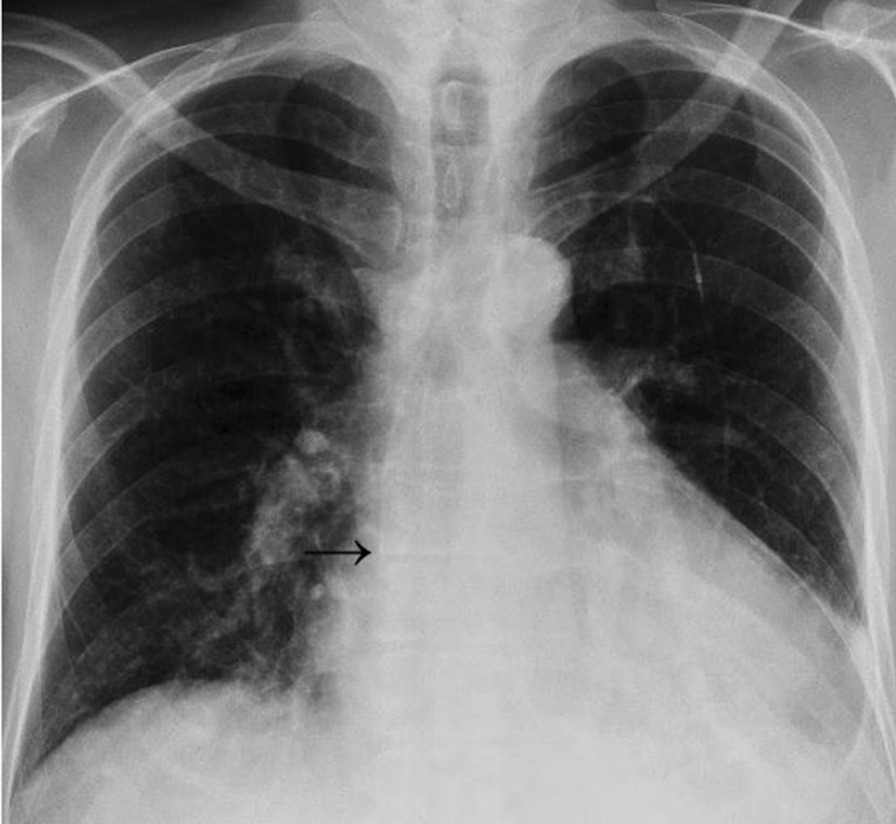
Postoperative chest X-ray. Chest fluoroscopy after surgery showing the correct localization of the catheter tip (arrow) at the superior vena cava/right atrium junction
